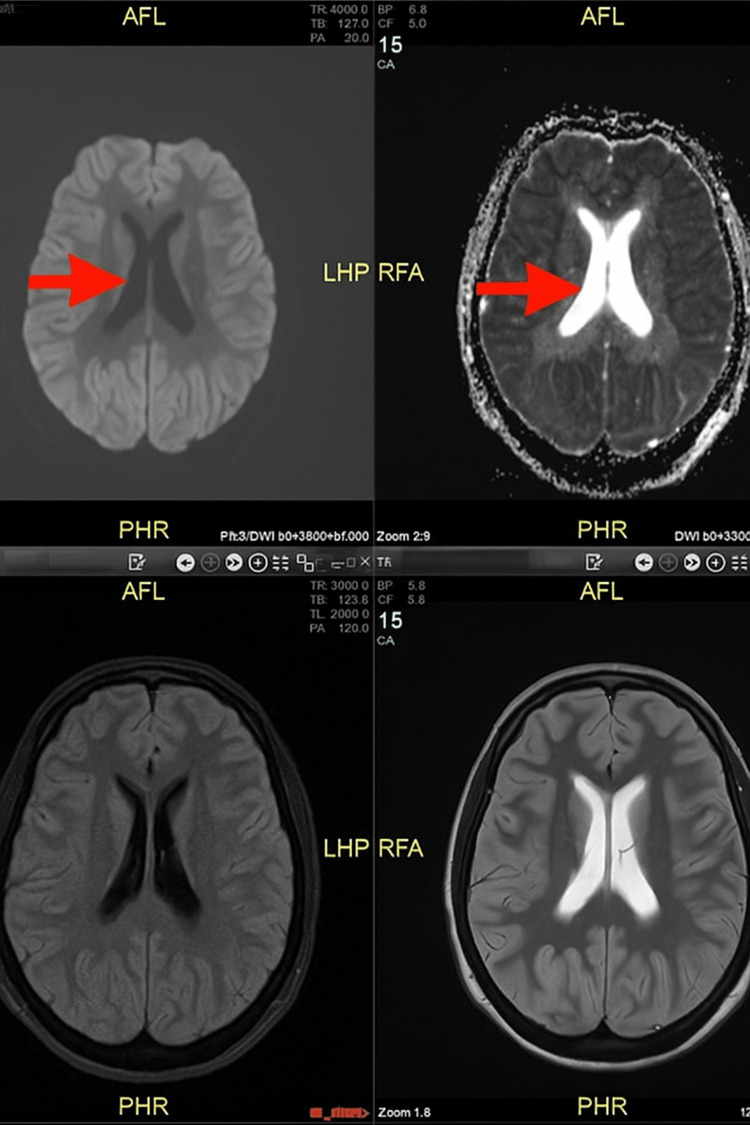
Magnetic resonance imaging (MRI) of the brain. MRI of the brain demonstrated widespread ischemic changes involving the cerebral cortex, with subtle but definite restricted diffusion, likely secondary to hypoxia.
